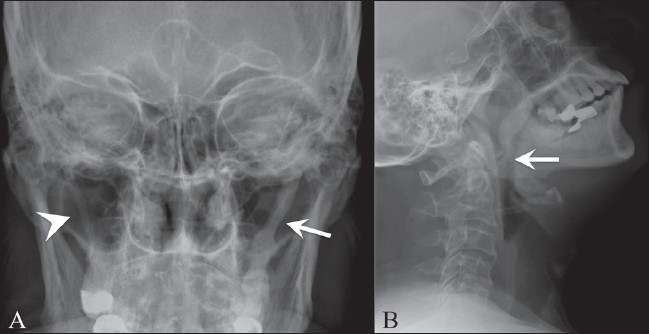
Antero-posterior (A) and lateral (B) radiographs of the skull show elongated styloid processes on both sides; there is a completely calcified stylohyoid ligament on the left side (arrow) and a partially calcified one on the right side (arrowhead)
